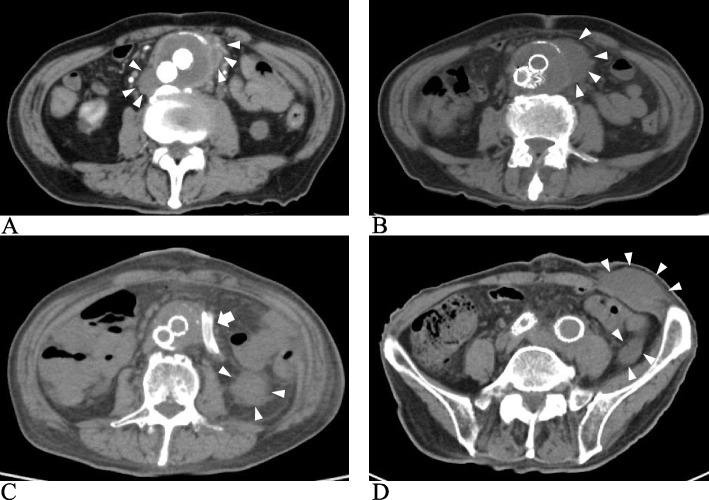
The results of computed tomography scan. A Before initiating antimicrobial therapy. B After 14 weeks of antibiotic therapy, periaortic fluid pooling became larger than that before antibiotics administration. C-D Three days after drainage surgery, the abscess expanded and spread along the drainage tube and formed a subcutaneous abscess around the drain insertion site. White arrowheads represent the abscess and white arrows represent the drainage tube
